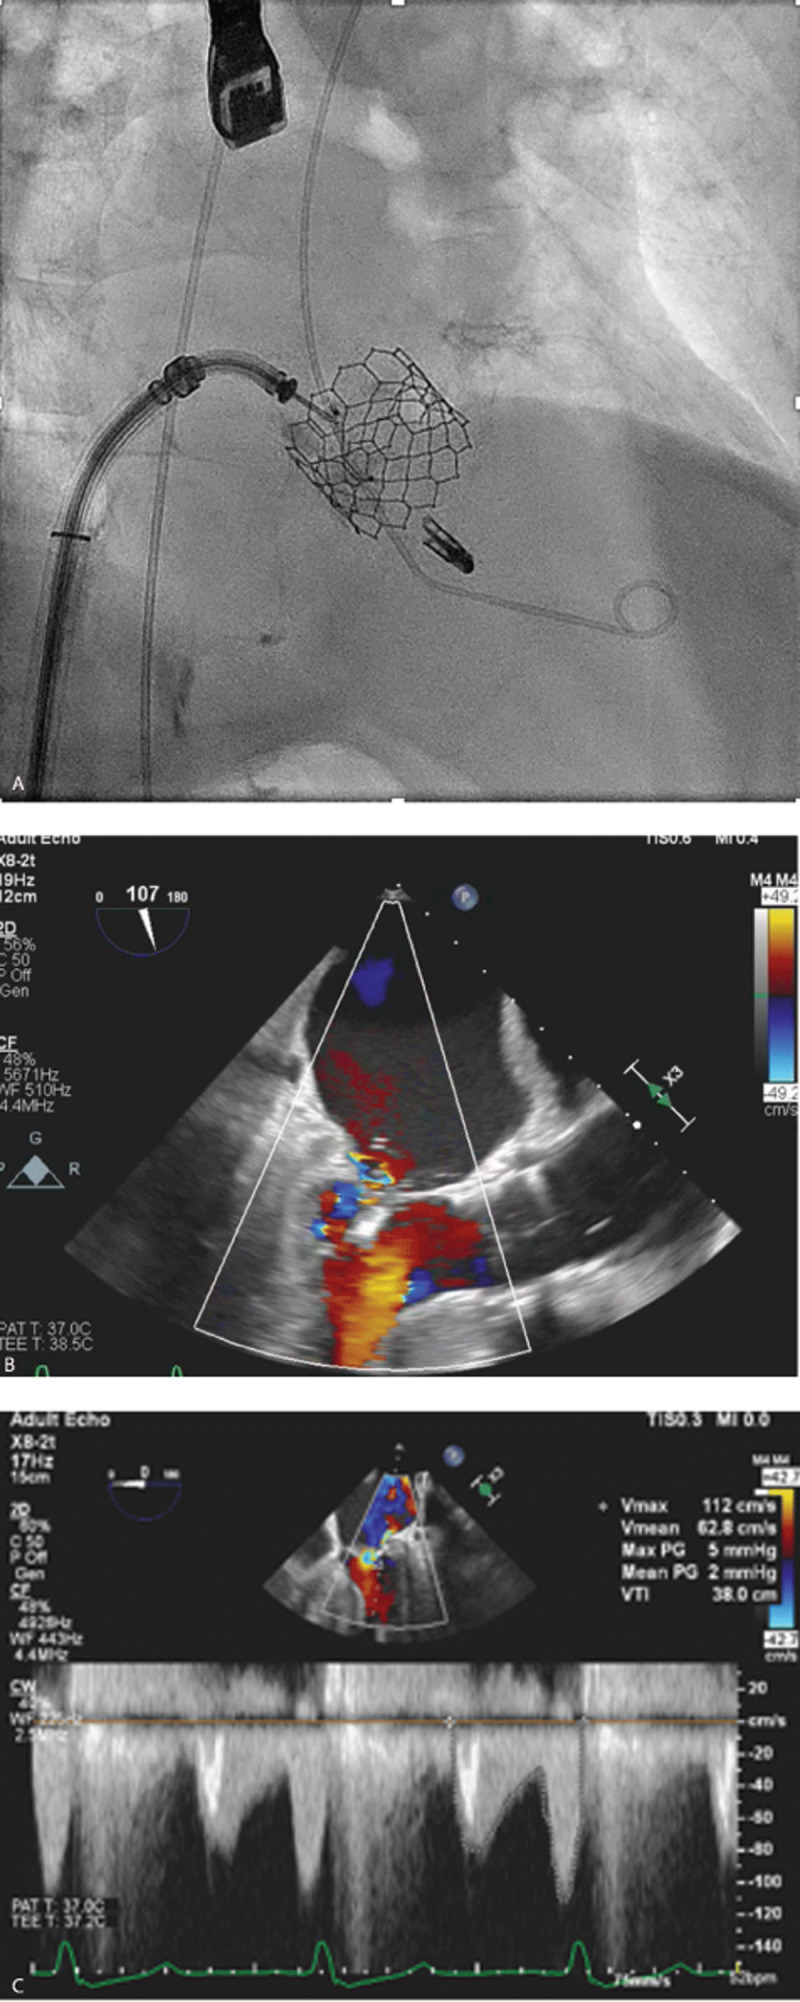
(A) Deployed MitraClip and (B, C) improved mitral regurgitation on transesophageal echocardiographic views.
